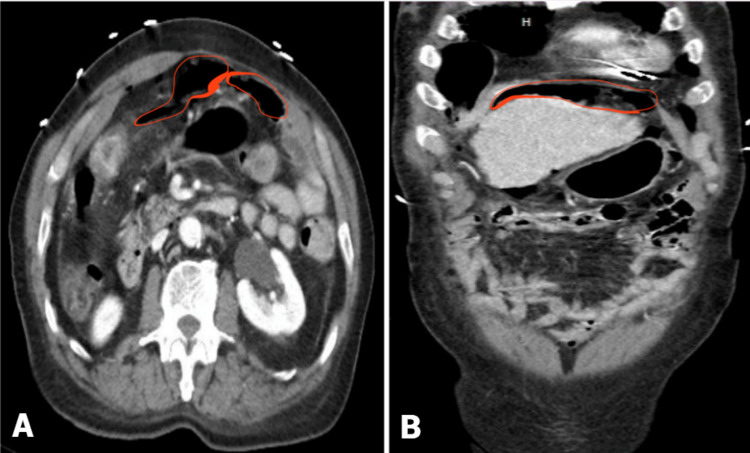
CT of the abdomen and pelvis with intravenous contrast A: transverse view, B: coronal view showing moderate volume pneumoperitoneum with trace free fluid in the upper abdomen (red marked areas) compatible with perforation
